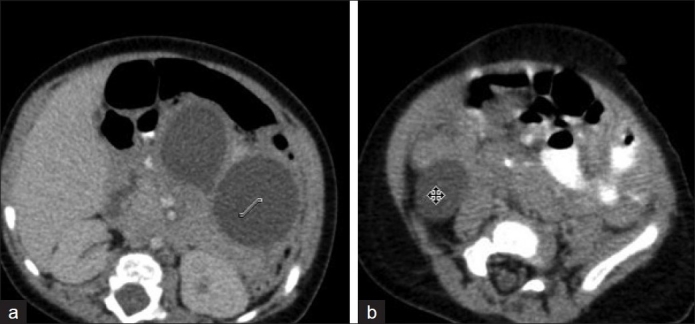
CT scan. (a) Dumbbell-shaped retrogastric cyst. (b) Separate cyst in the right iliac fossa
